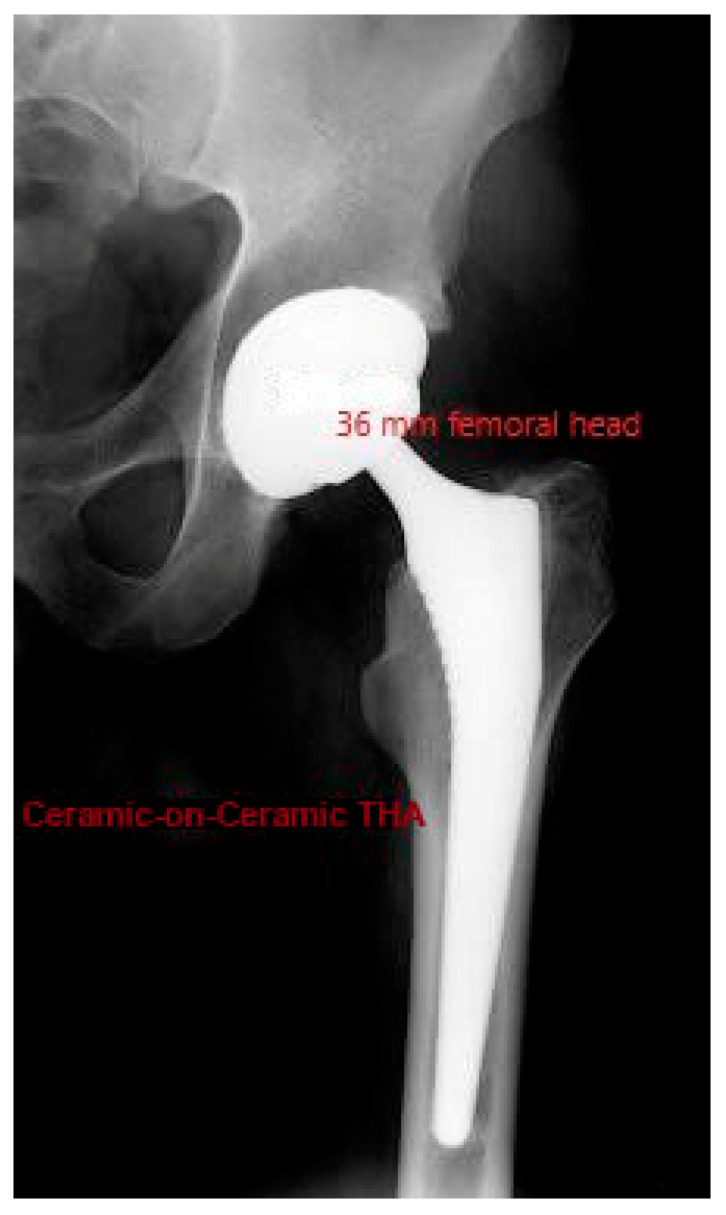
X-ray of ceramic-on-ceramic total hip arthroplasty. A 36 mm femoral head implant was used.
